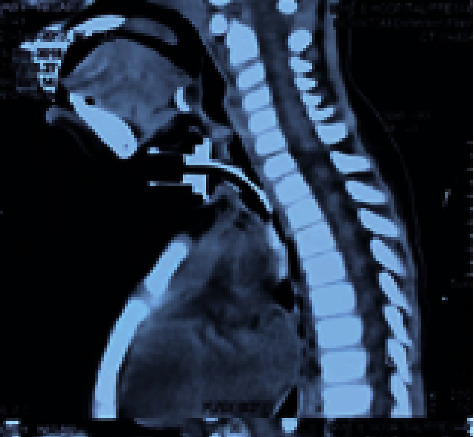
Postoperative CT image showing adequate subglottic airway lumen.
